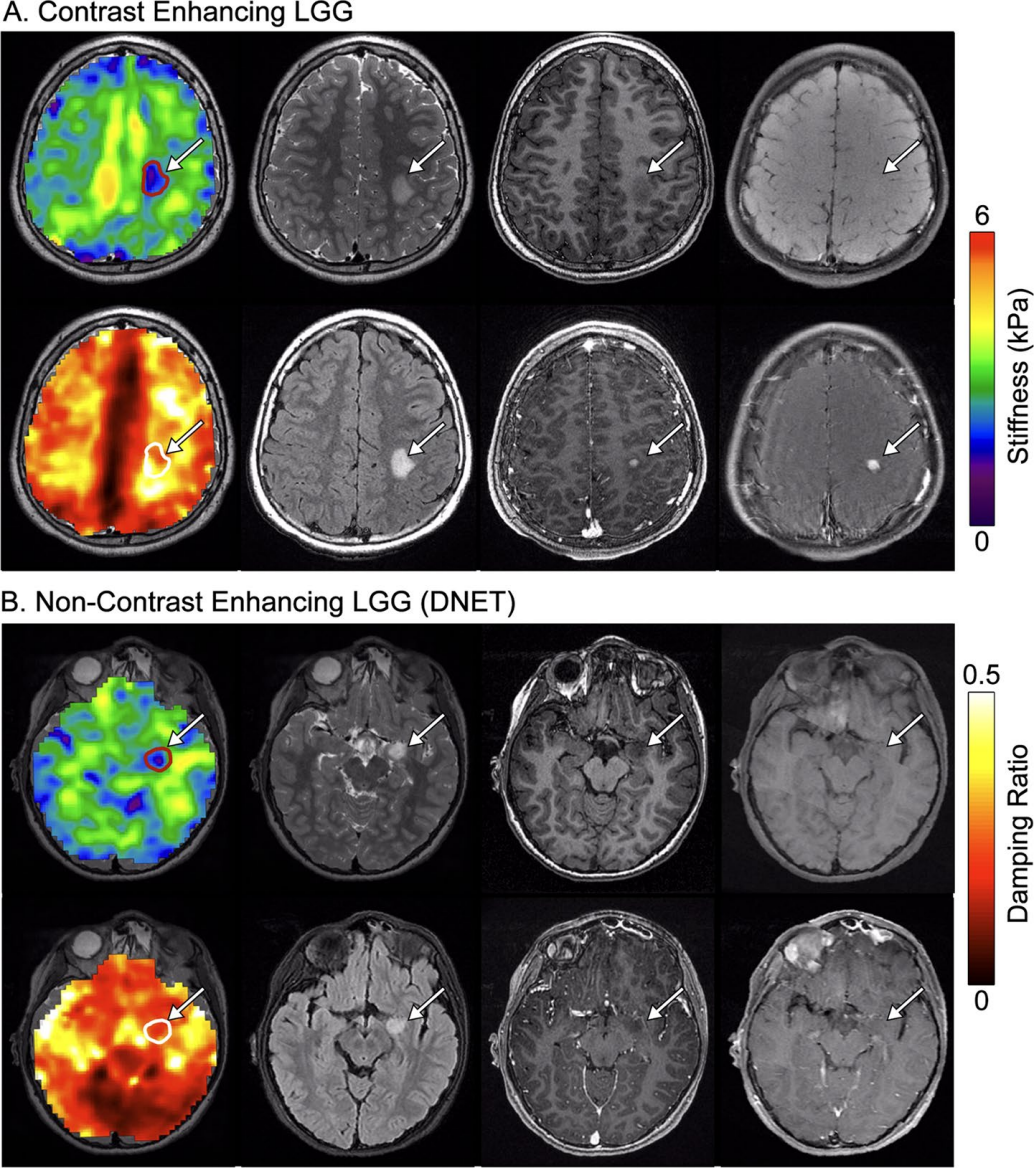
Both contrast enhancing and non-contrast enhancing LGGs appear soft. (A) Patient 11, 11-year-old male, with a contrast enhancing low-grade glioma and focal seizures. (B) Patient 2, 9-year-old female, with a non-contrast enhancing left temporal lobe dysembryoplastic neuroepithelial tumor (DNET). Top rows, left-to-right: Stiffness map, T_2_-CUBE, T_1_-BRAVO, MEMP; Bottom rows, left-to-right: Damping ratio map, T_2_-FLAIR, post contrast T_1_-BRAVO, post contrast MEMP
